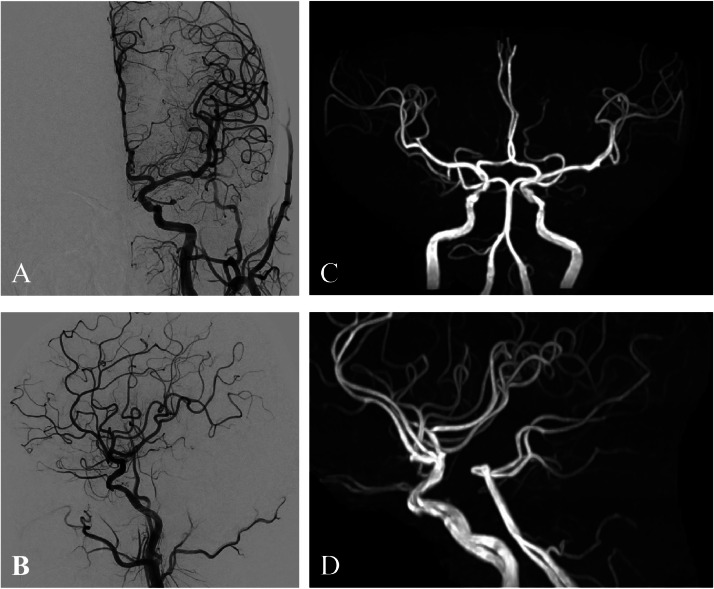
(A,B) Postoperative DSA of the left ICA showing that the DAVF has disappeared. (C,D) The disappearance of the DAVF is confirmed on follow-up MRI.
